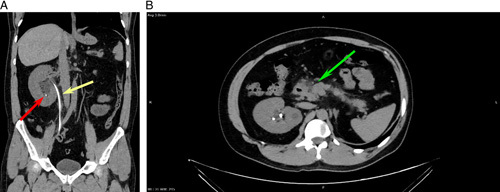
A, Coronal section CT KUB, shows JJ stent in right ureter ‘yellow arrow’, still there is mild pelvicalyceal dilation, small stone in lower pole calyx ‘red arrow’. B, Axial section of CT KUB, shows edema and fat stranding between the head of the pancreas and the second part of the duodenum, with a feature of acute pancreatitis.
